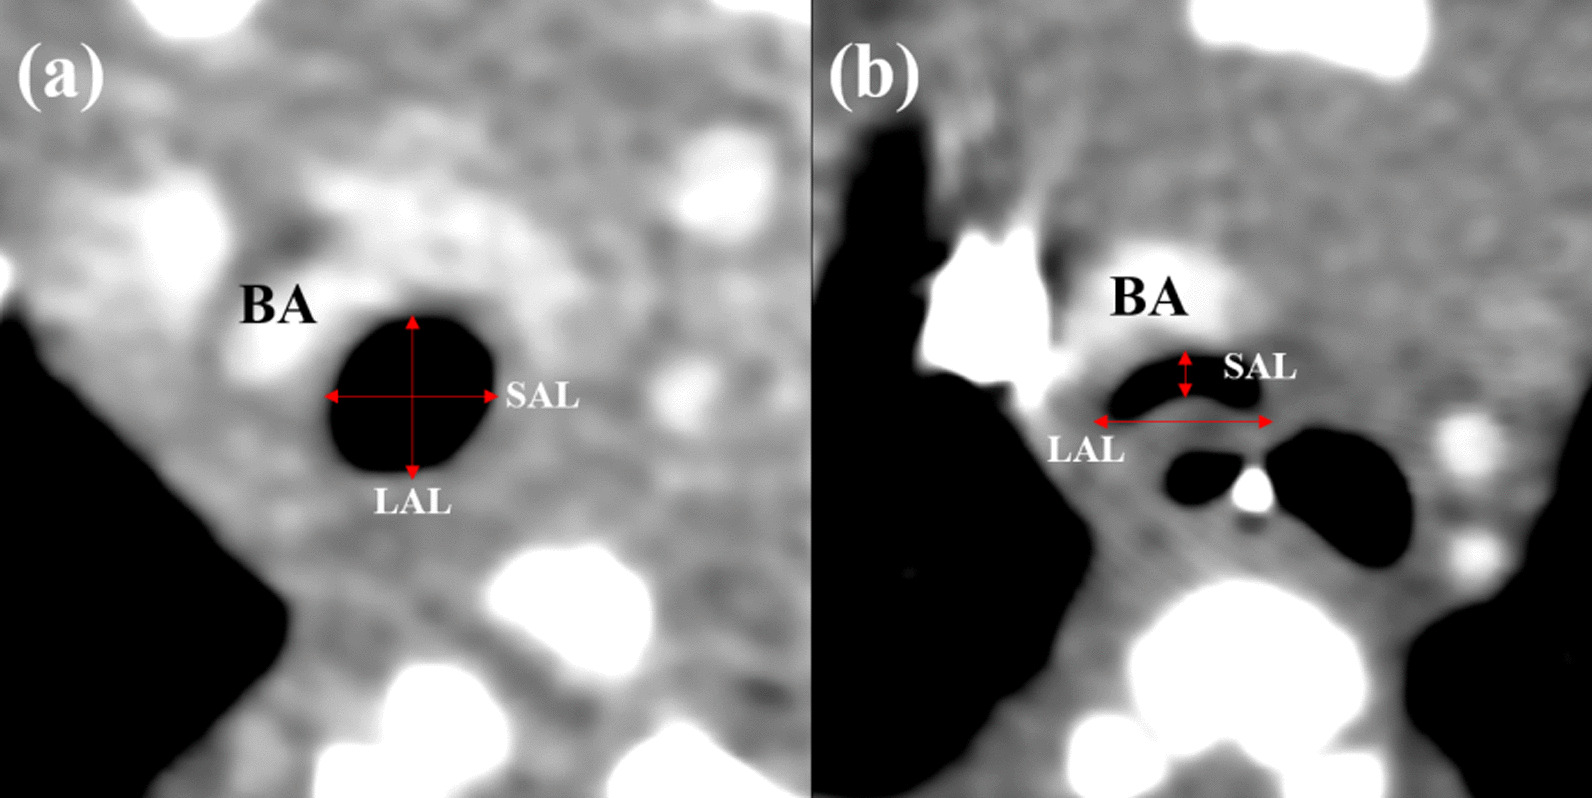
Computed tomography images indicating the calculation of LAR. At the level where the brachiocephalic artery crosses the trachea, LAR is calculated by dividing the long axis length of the trachea by the short axis length. a Neonate without tracheal collapse. b Neonate with tracheal collapse complicated by esophageal atresia. *BA* brachiocephalic artery, *LAL* long axis length of the trachea, *SAL* short axis length of the trachea
